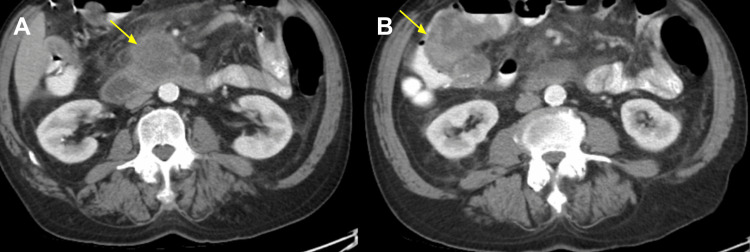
CT Abdomen Pelvis With and Without Contrast (A) Uncinate process mass abutting the duodenal C-loop with narrowing of the superior mesenteric vein (SMV), measuring 6.9 x 5.2 cm. (B) Right hemicolectomy anastomosis with a filling defect concerning tumor recurrence, measuring 7.4 x 6.6 cm.
